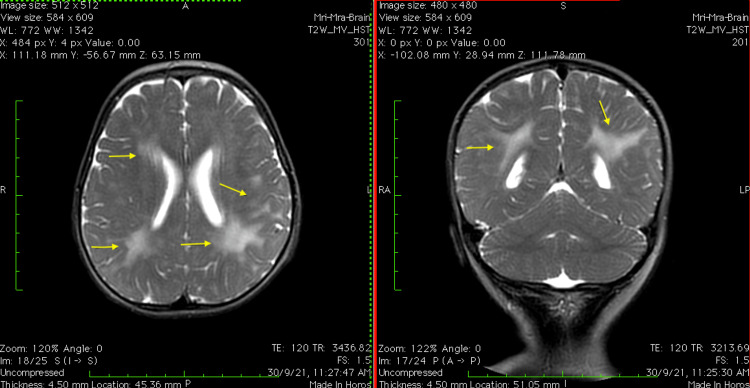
The patient's brain MRI Increased signal intensity on T2 and FLAIR and low signal intensity on T1 are seen in the periventricular white matter symmetrically, especially posteriorly, with extension to the subcortical white matter, especially parietally FLAIR: fluid-attenuated inversion recovery
